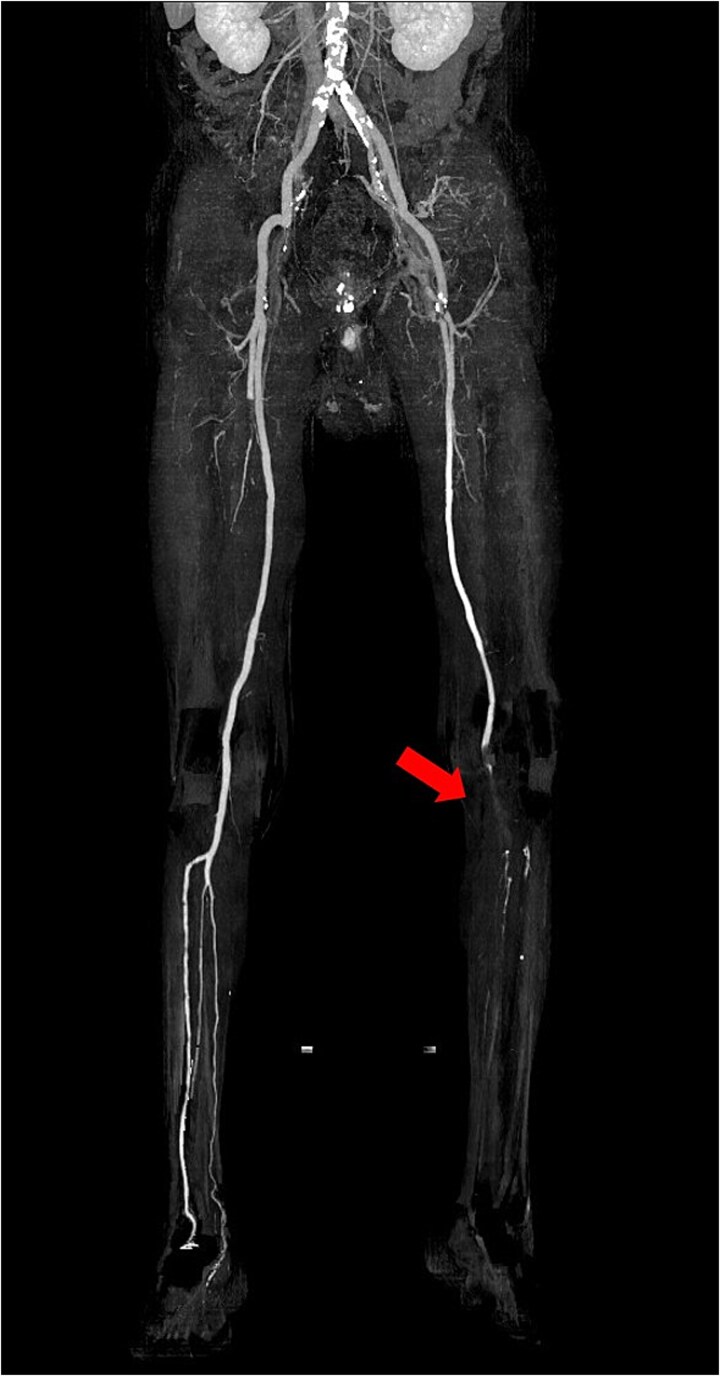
Preoperative 3D reconstructed contrast-enhanced CT. No contrast effect is seen in the blood flow of the lower leg.
